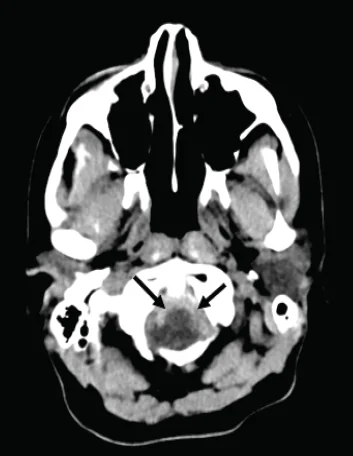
Computed tomography of the head (axial view) at the level of the upper cervical spine showing hemorrhage into the spinal canal (arrows).
